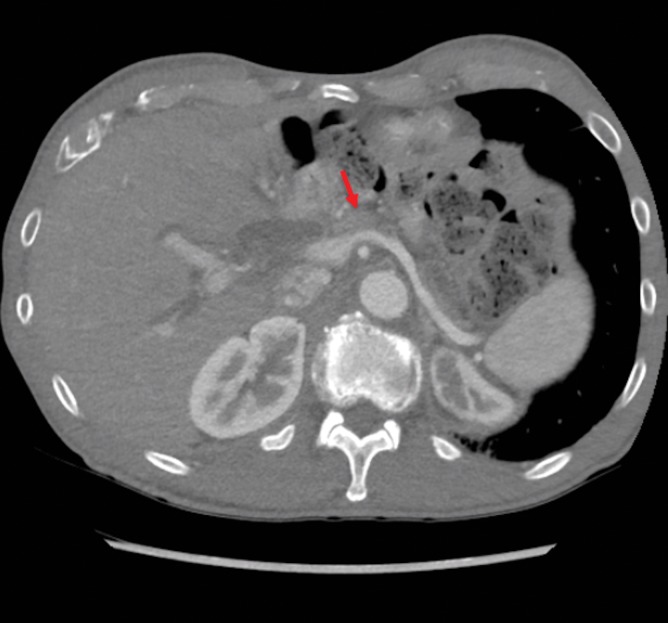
CT image revealing a pancreatic neck mass (arrow) measuring 2.2 × 1.3 cm, with dilated intrahepatic bile ducts and patent splenic vein and portal vein. CT, computed tomography.
